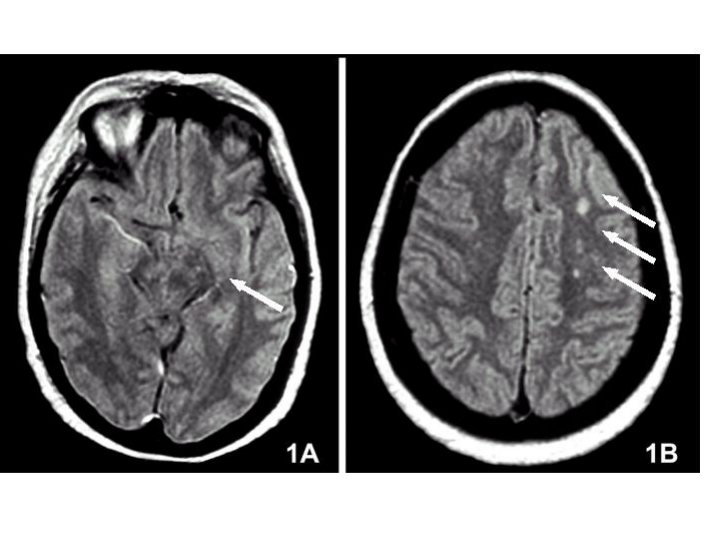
Magnetic resonance imaging scan showing multiple ischemic lesions in left cerebral peduncle (1A) and semioval centres (1B).
